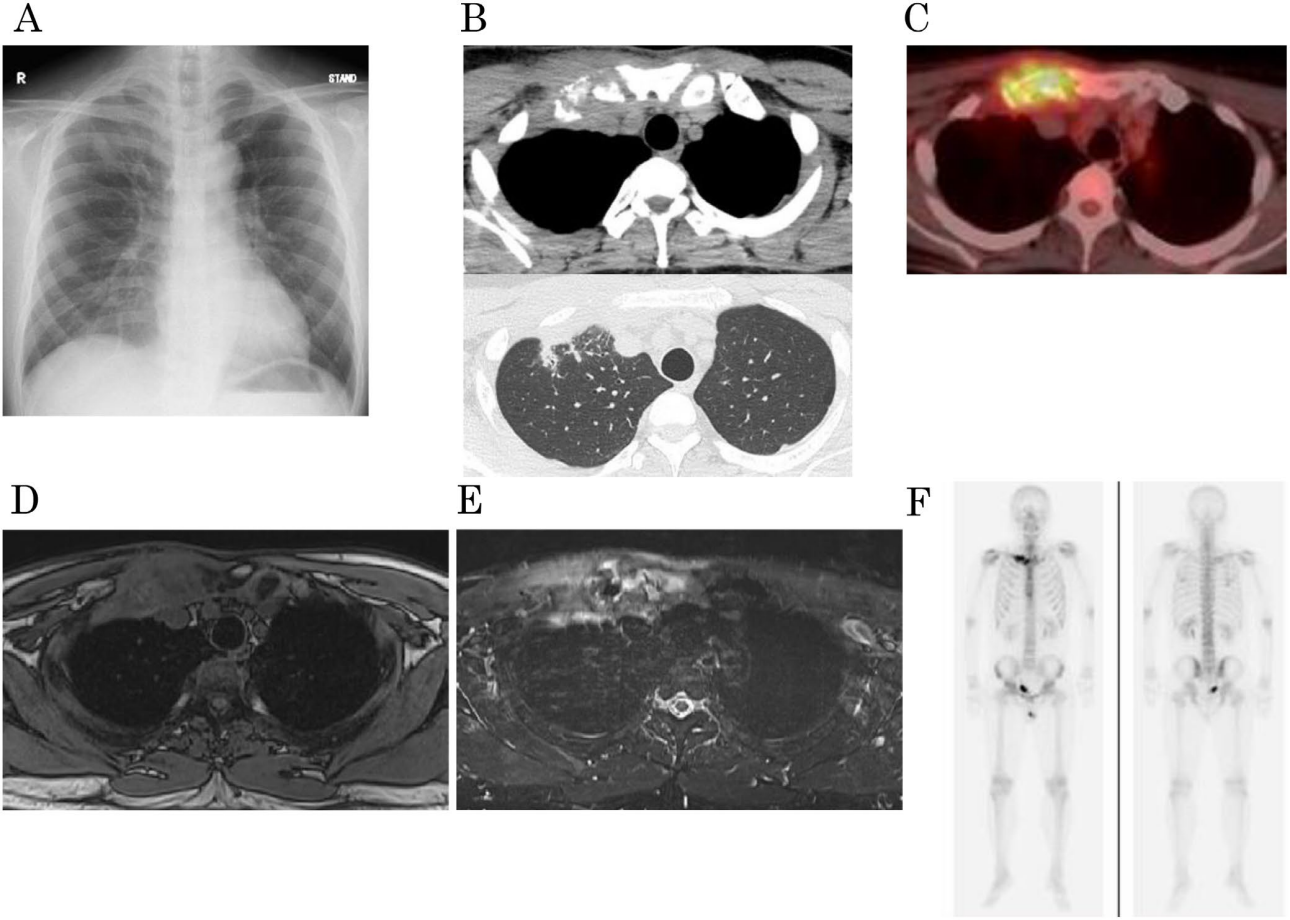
Radiographic examinations. (A) Chest X‐ray. (B) Chest computed tomography (CT). (C) Fluorodeoxyglucose positron emission CT. (D) Chest Magnetic Resonance Imaging (MRI) (T1‐weighted). (E) Chest MRI (T2‐weighted). (F) Techbetium‐99 m bone scintigraphy.
